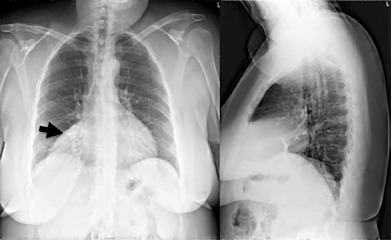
Chest radiograph: Anterior-posterior and lateral films demonstrating anterior mediastinal opacity over the right heart border (arrow).
